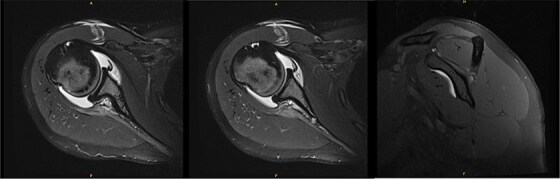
Initial MRI arthrogram revealed low-grade undersurface fraying of the supraspinatus and infraspinatus tendons consistent with internal impingement, hypertrophic posterior inferior labrum without visible tearing, and no evidence of paralabral cyst.
